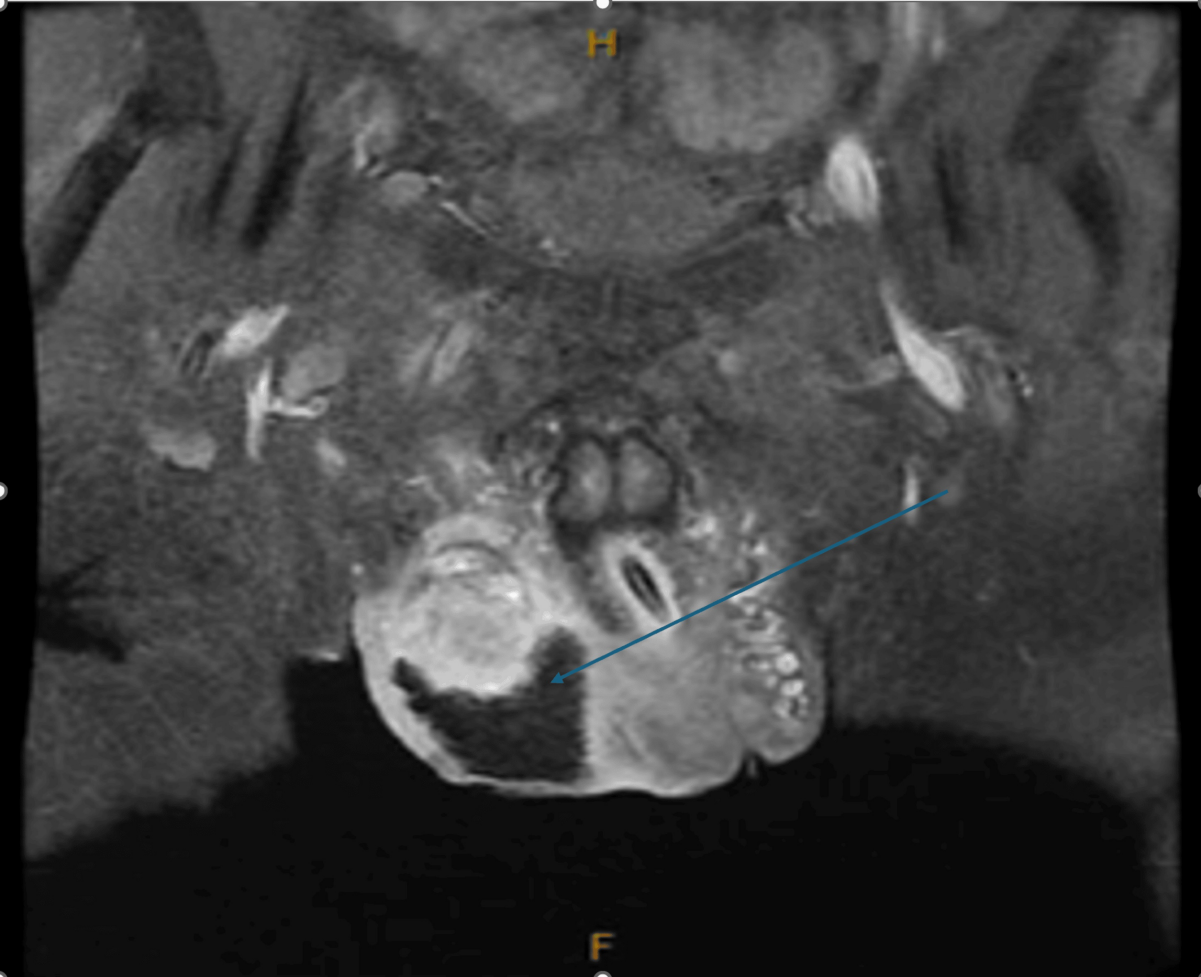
Magnetic resonance imaging on day 1 post-scrotal drainage The arrow indicates some remaining micro-collections/abscesses.
